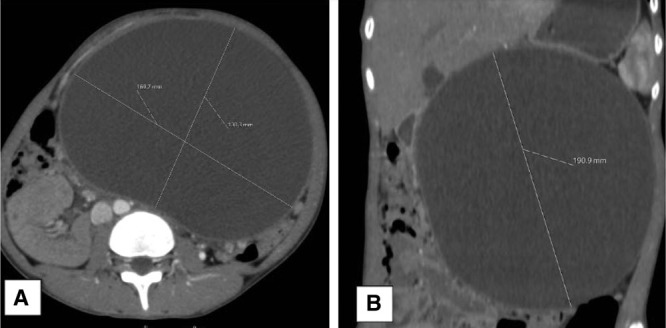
Abdominal CT scan with contrast media at the arterial phase, in axial section (A) and coronal reconstruction (B) showing a large retroperitoneal cystic mass related to a pancreatic pseudocyst. Note the right side repression of the retroperitoneal vessels.
